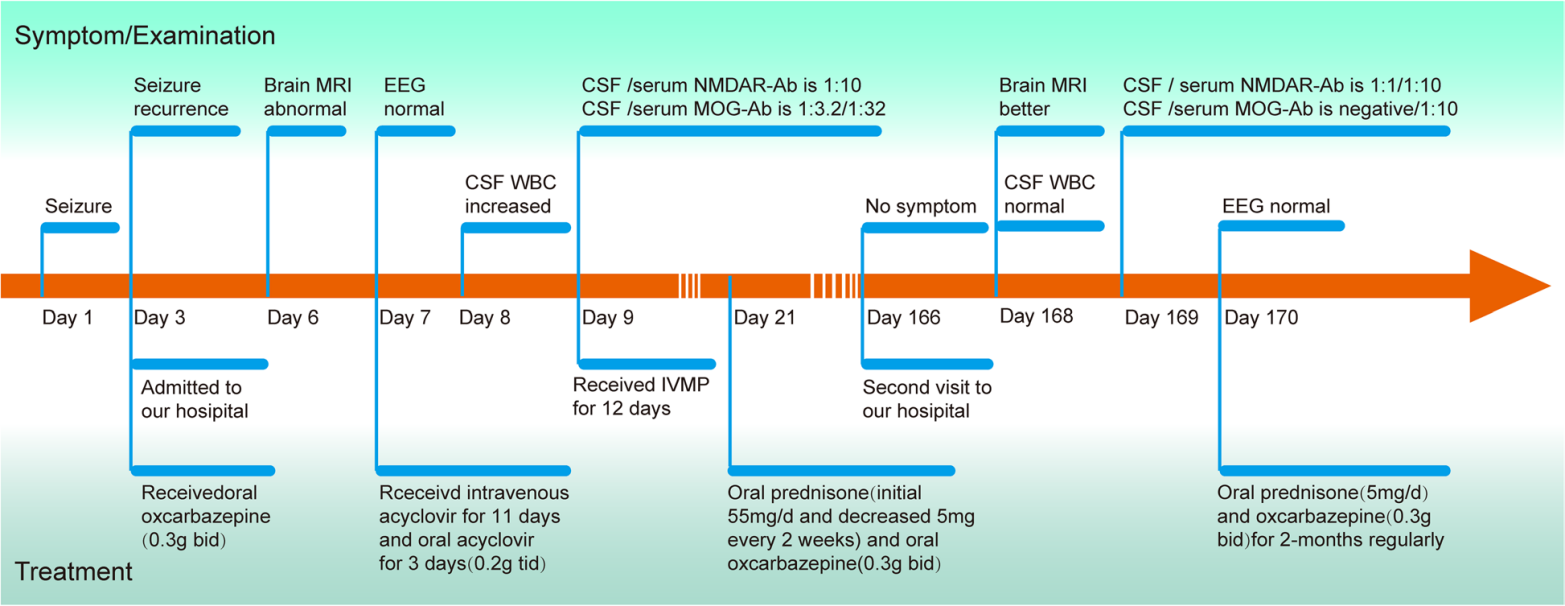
Timeline with clinical manifestation, treatment progression, and diagnosis time. EEG: Electroencephalograph; MRI: Magnetic Resonance Imaging; IVMP: Intravenous methylprednisolone; Abs, antibodies; WBC: white blood cell; CSF: cerebrospinal fluid; NMDAR: Anti-N-methyl-D-aspartate receptor; MOG: Myelin oligodendrocyte glycoprotein
